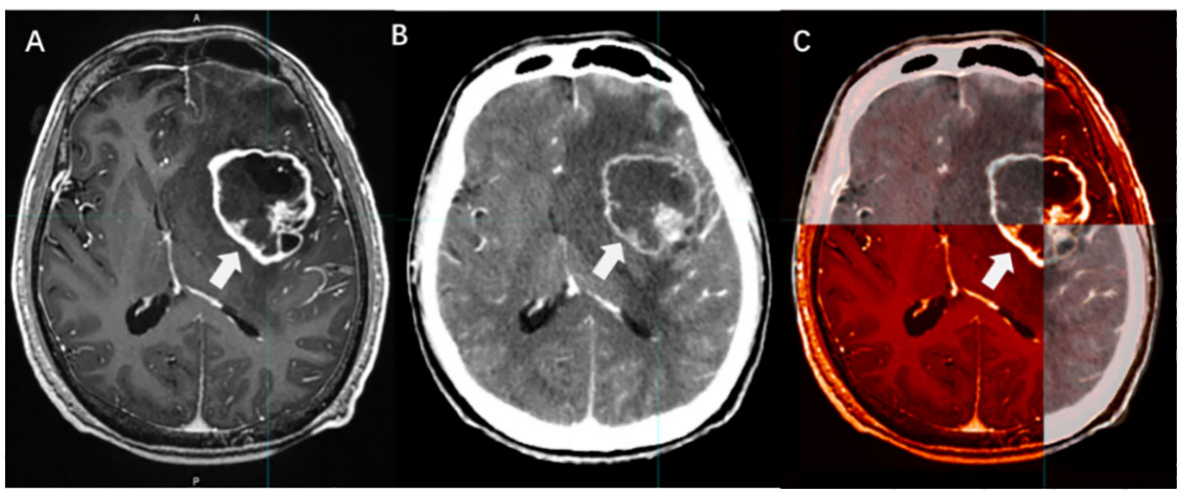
The boundary determination of BMs applying CT/MRI fusion. [(A) CE-T1WI images; (B) Enhanced CT images; (C) CT/MRI fusion].
